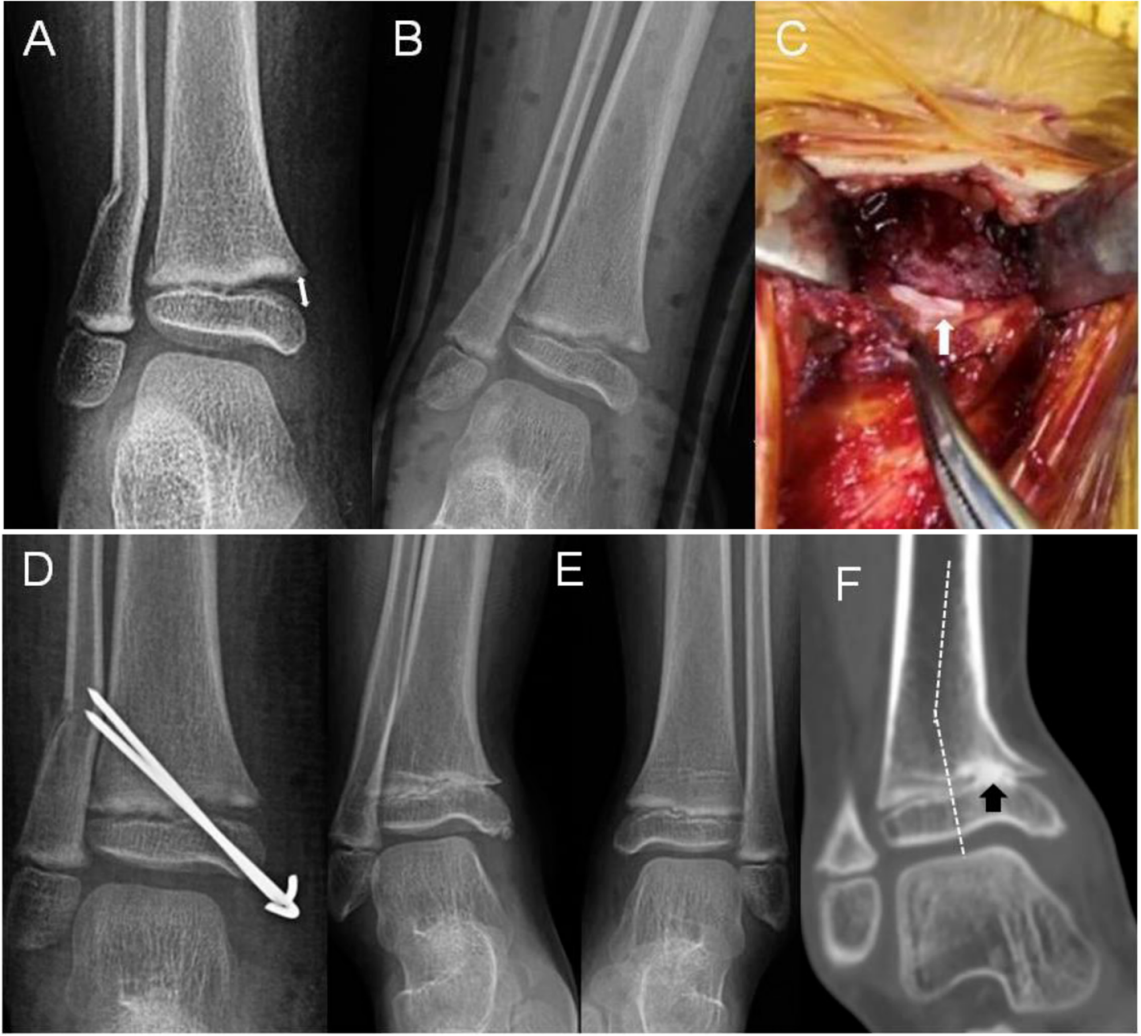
A 3.9-year-old girl. a AP radiograph showed 7 mm displacement of the fracture (white double arrow). b AP radiograph obtained 8 days after closed reduction showed the significant residual gap. c Interposed periosteum was shown after open reduction (white arrow). d AP radiograph demonstrated the reduction immediately post operatively. e AP radiograph of bilateral ankles obtained 14 months after treatment showed a partial premature arrest along the medial tibial physis. f Coronal view detected the bone bridge (black arrow) and a varus deformity of 15 degrees (white dashed line)
